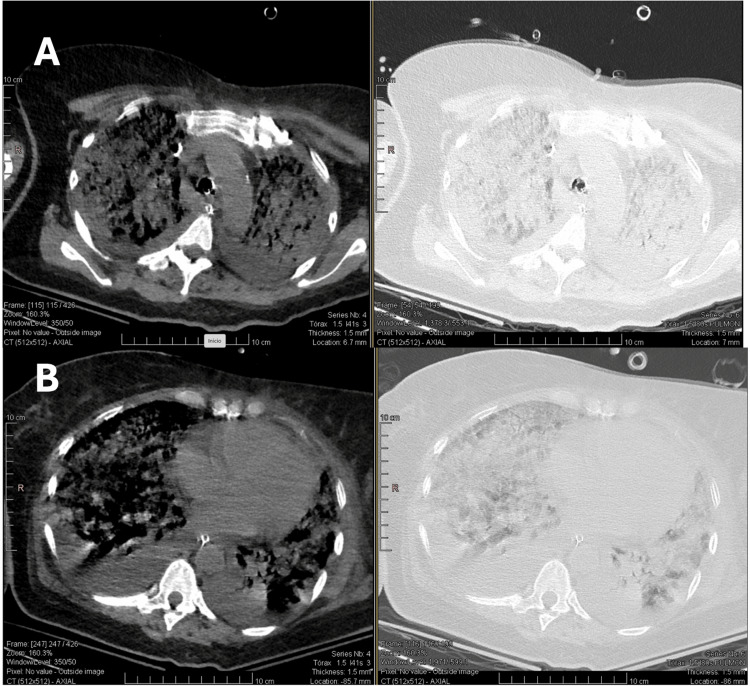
Chest computed tomography 1 day prior to the initiation of VV-ECMO therapy demonstrating diffuse bilateral infiltrates consistent with severe ARDS. (A) Axial image at the level of the aortic arch. (B) Axial image at the level of the atria. VV-ECMO: veno-venous extracorporeal membrane oxygenation; ARDS: acute respiratory distress syndrome
